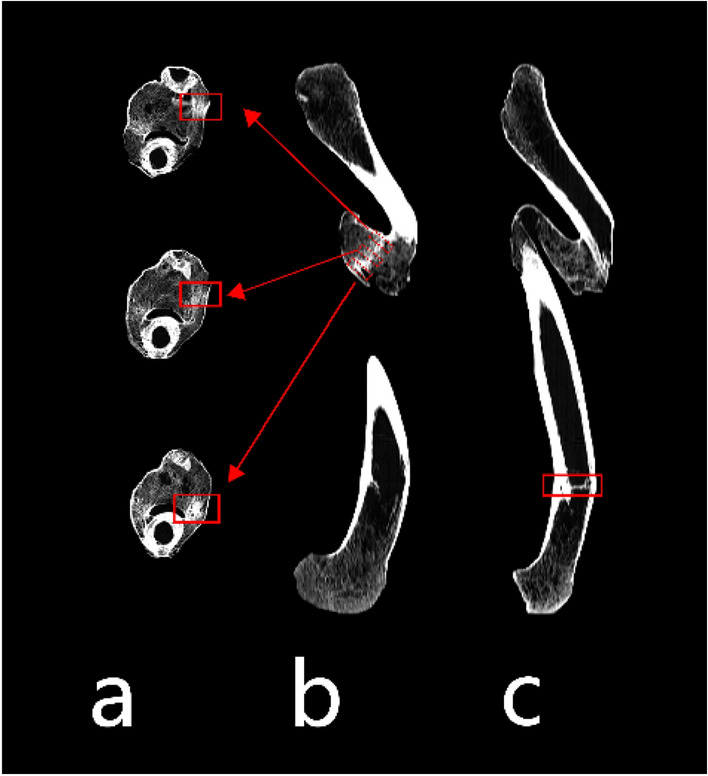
CT image of the femur. (a and b) show the proximal fracture segment callus and bundles of pressure trabeculae; (c) shows the distal impacted fracture segment with an intramedullary callus.
